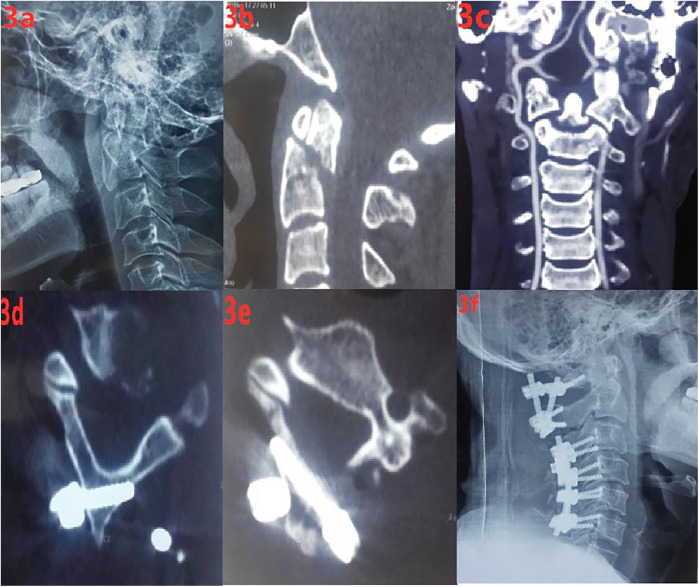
(a) preoperative cervical lateral x-ray; (b,c) preoperative cervical spine CT; (d,e) postoperative CT showing that the C2 laminar screws were in good position and did not protrude into the spinal canal; (f) postoperative lateral x-ray of the cervical spine.
